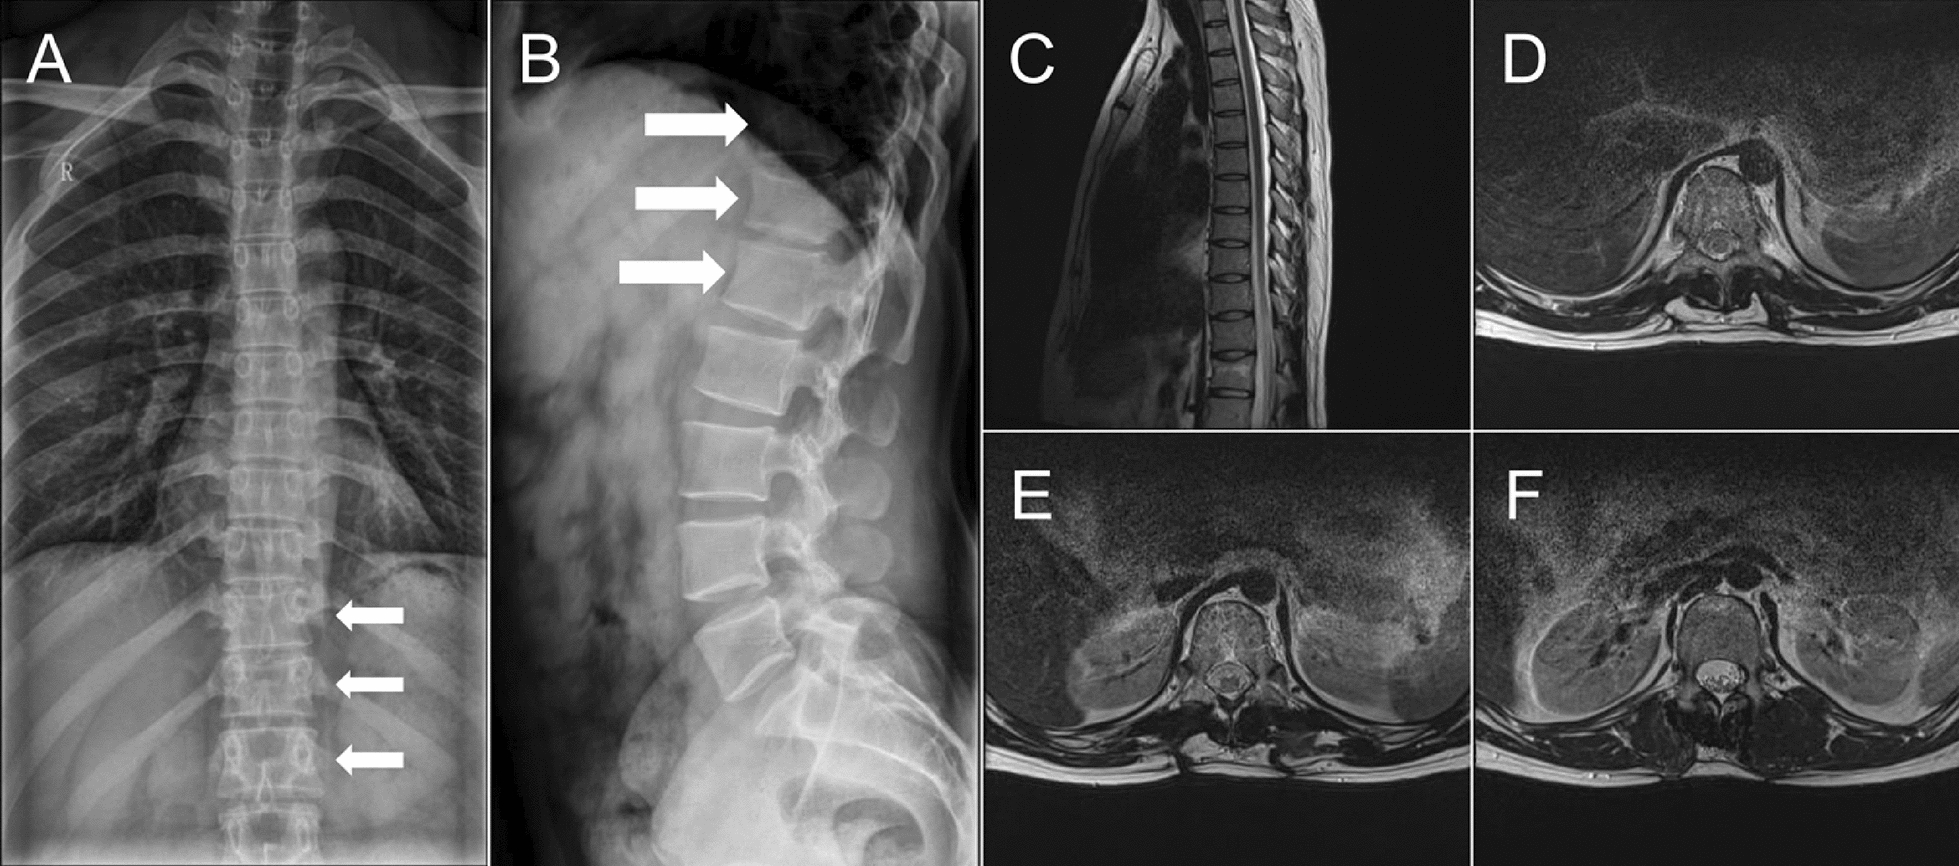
Long-term imaging at 2.5 years post-injury demonstrates complete osseous integration and adaptive muscle recovery. A, B Axial and sagittal radiograph images show that the previous pedicle screw channels have fully ossified, with no remaining radiolucent tracks (arrows). C A sagittal T2-weighted magnetic resonance imaging slice reveals robust hypertrophy of the paraspinal musculature surrounding the once-compromised T12 level. D–F Serial axial T2-weighted magnetic resonance imaging sections through the T12 area confirm circumferential regeneration and thickening of the paraspinal muscles encircling the stabilized segment, correlating with the patient’s functional recovery
